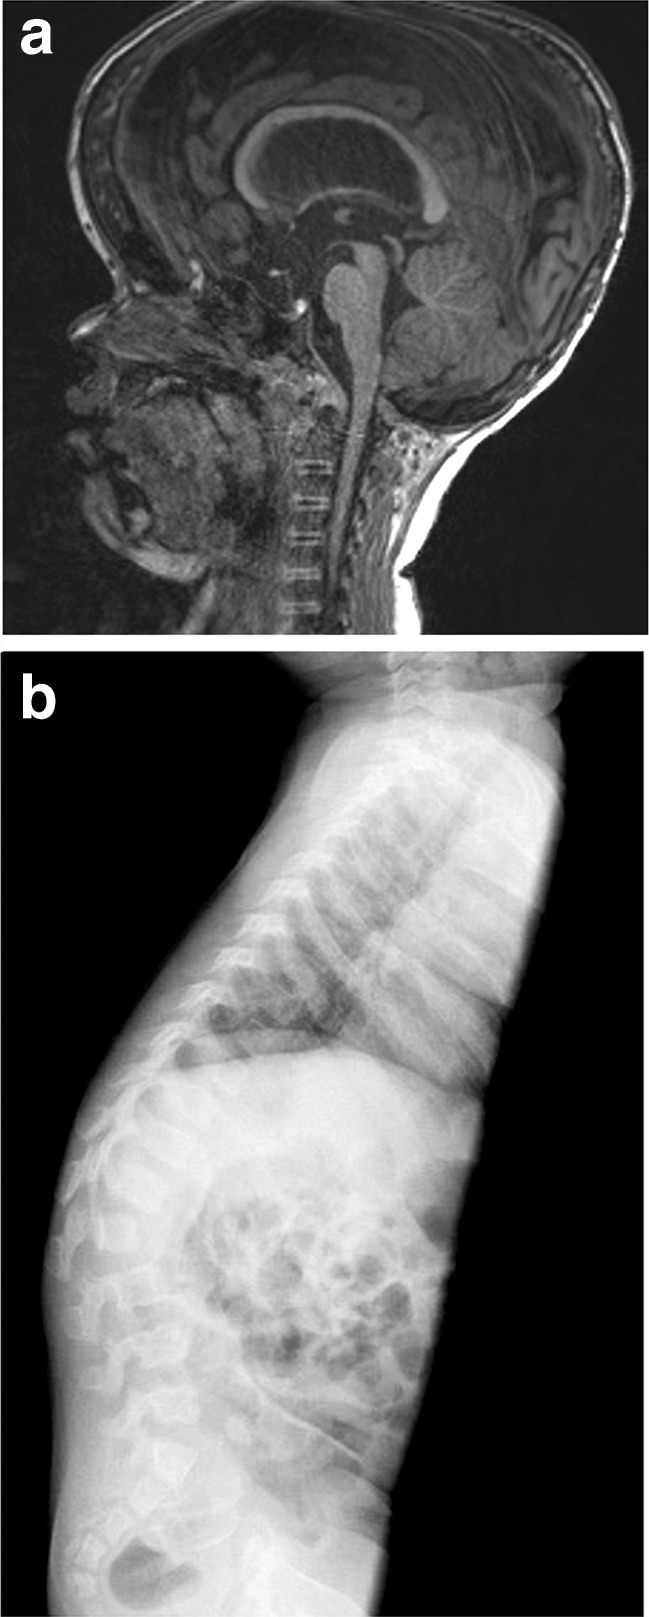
a MRI image of a four and a half year old boy showing a narrow foramen magnum. This finding is seen in almost all children with achondroplasia and is not by itself an indication to perform neurosurgery. b X-ray showing the typical configuration of the lumbar spine in achondroplasia with shortening in the AP diameter and scalloping if the posterior endplates. More significant shortening of the first lumbar vertebra is also a risk factor for developing a fixed kyphosis and would be an indication for treatment by bracing
